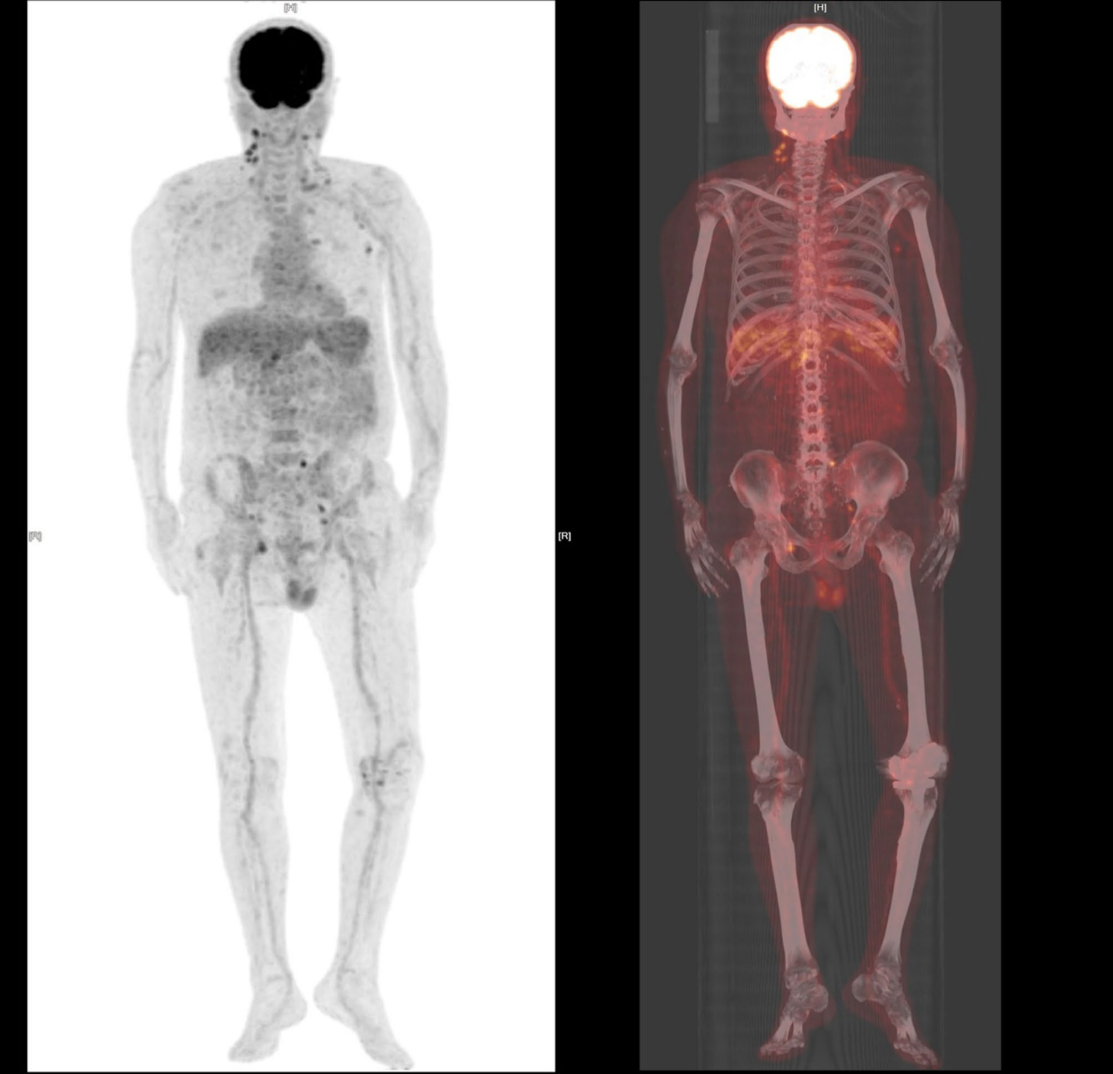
PET/CT Imaging of Whole-Body. (1) Postoperative bladder malignancy: The bladder shows reduced volume with low metabolic activity, with no definitive signs of tumor recurrence; (2) Multiple lymph nodes in the hepatic-gastric space, porta hepatis, bilateral inguinal regions, bilateral carotid sheath, clavicular, and bilateral axillary areas: Some of these lymph nodes are slightly enlarged with mild to moderate metabolic increase, which is more consistent with reactive lymphadenopathy; (3) Bilateral renal atrophy with faint imaging findings; (4) Age-related cerebral changes; (5) Multiple chronic inflammatory and calcified foci in both lungs, with small amounts of pleural effusion on both sides; (6) Multiple calcific lesions observed in the aortic valve, mitral valve, bilateral coronary arteries, thoracoabdominal aorta, and some branches; (7) Post bilateral knee joint replacement: Mildly increased metabolic activity in the synovial membranes; increased metabolism at the attachment site of the right ischial tuberosity and obturator internus muscle, suggesting inflammatory uptake
